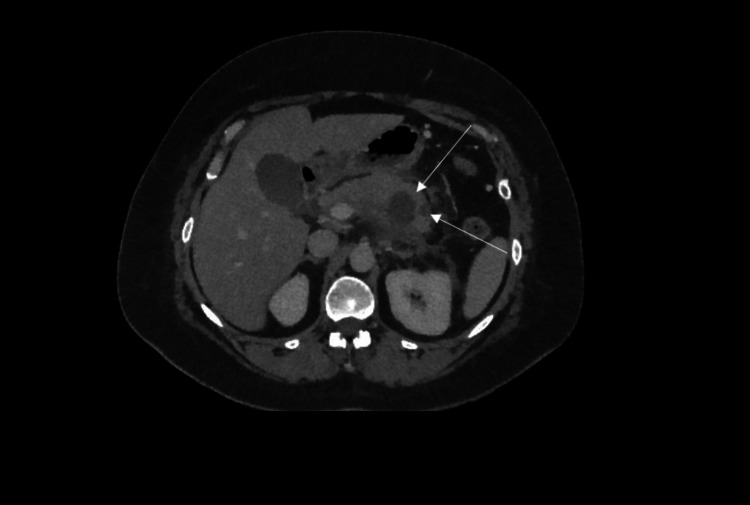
CT abdomen showing acute pancreatitis with peri-pancreatic inflammatory stranding and two pseudocysts in the tail of the pancreas.
